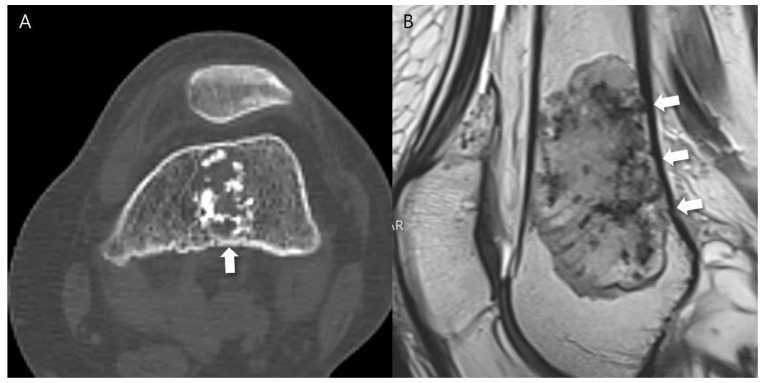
Extensive endosteal scalloping ≥ 2/3 of the lesion length in a patient with ACT in the distal femur. (A) An axial CT image shows a lobulated mass containing chondroid matrix mineralization and focal endosteal scalloping (arrow). (B) A sagittal T2-weighted image shows a lobulated mass with heterogeneously increased signal intensity and extensive endosteal scalloping ≥ 2/3 of the lesion length (arrows).
